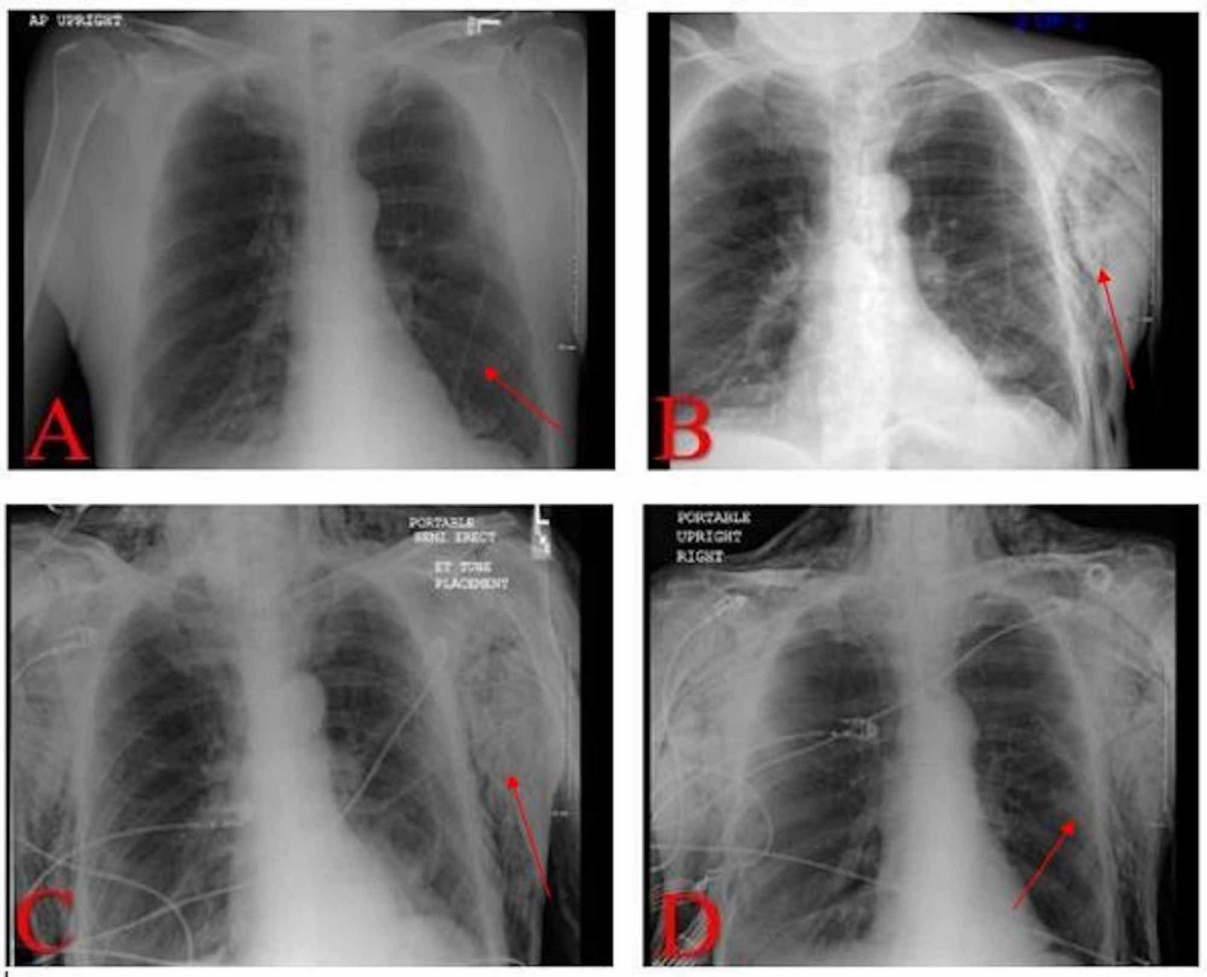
Series of chest X-rays of the patients (A) Chest X-ray on day 0 after failed biopsy. (B) Chest X-ray on day 1 after biopsy and day 3 after aborted biopsy: development of subcutaneous emphysema (arrow). (C) Chest X-ray on day 2 after biopsy and day 4 after failed biopsy, and rapid response was called and the patient was transferred to ICU for intubation: worsening subcutaneous emphysema (arrow), airway involvement. (D) Chest X-ray on day 2 after biopsy and day 4 after failed biopsy, with 24-French chest tube placement (arrow). ICU, intensive care unit
